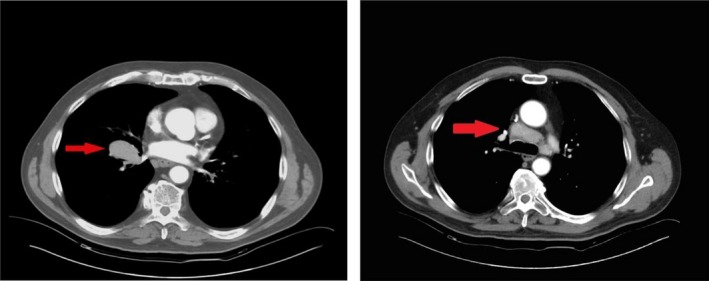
left panel: CT scan showing opacity of the right lower lobe; right panel: CT scan showing precarenal, paratracheal, subcarinal nodes N2.
